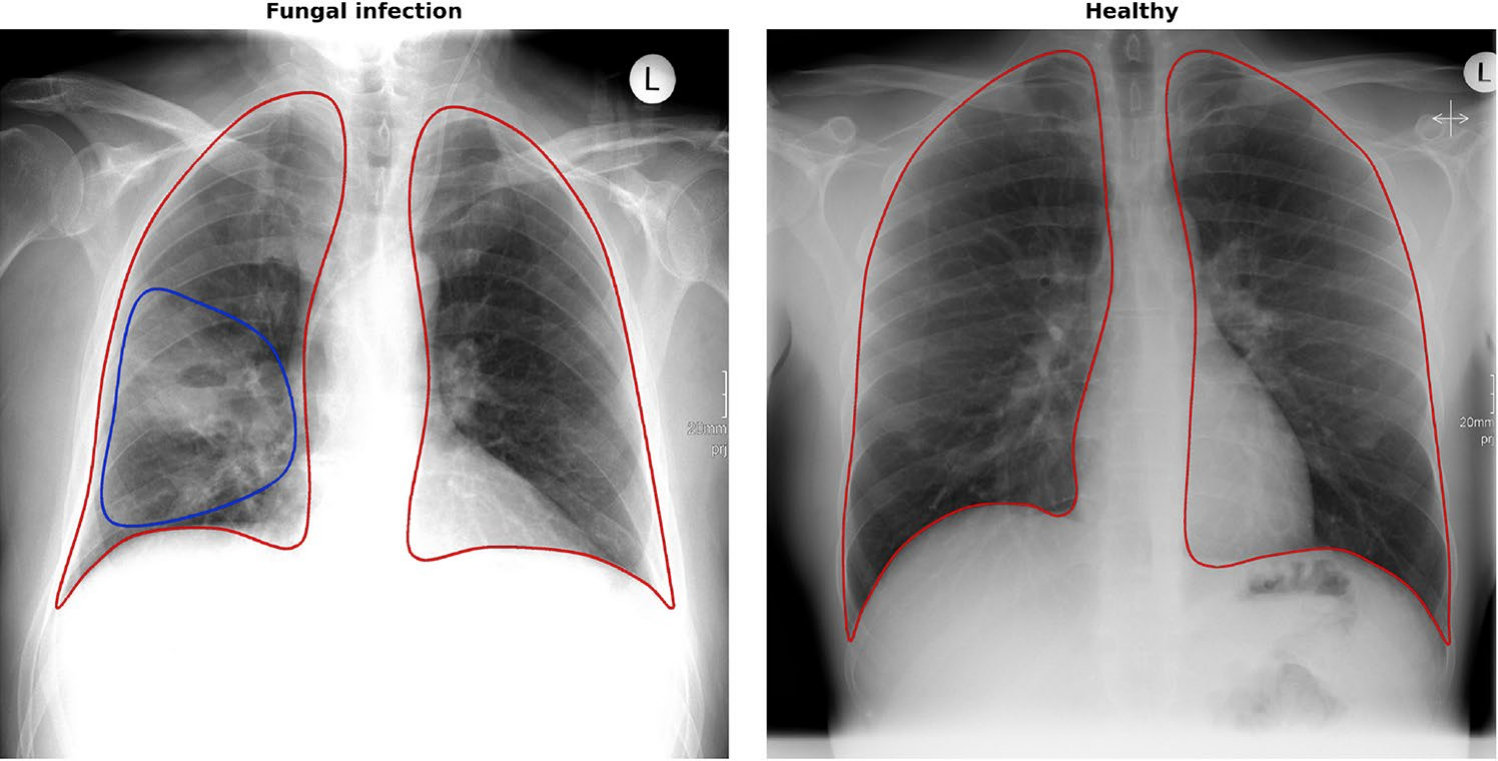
Chest X-ray ROI images with lungs marked in red. (Left) Fungal infection, typical ground-glass opacification marked in blue (ROI) All ROI annotations of this study show only the boundary of the infection, instead of a complete mask and use the same blue color regardless of pneumonia class. (Right) Healthy patient image.
